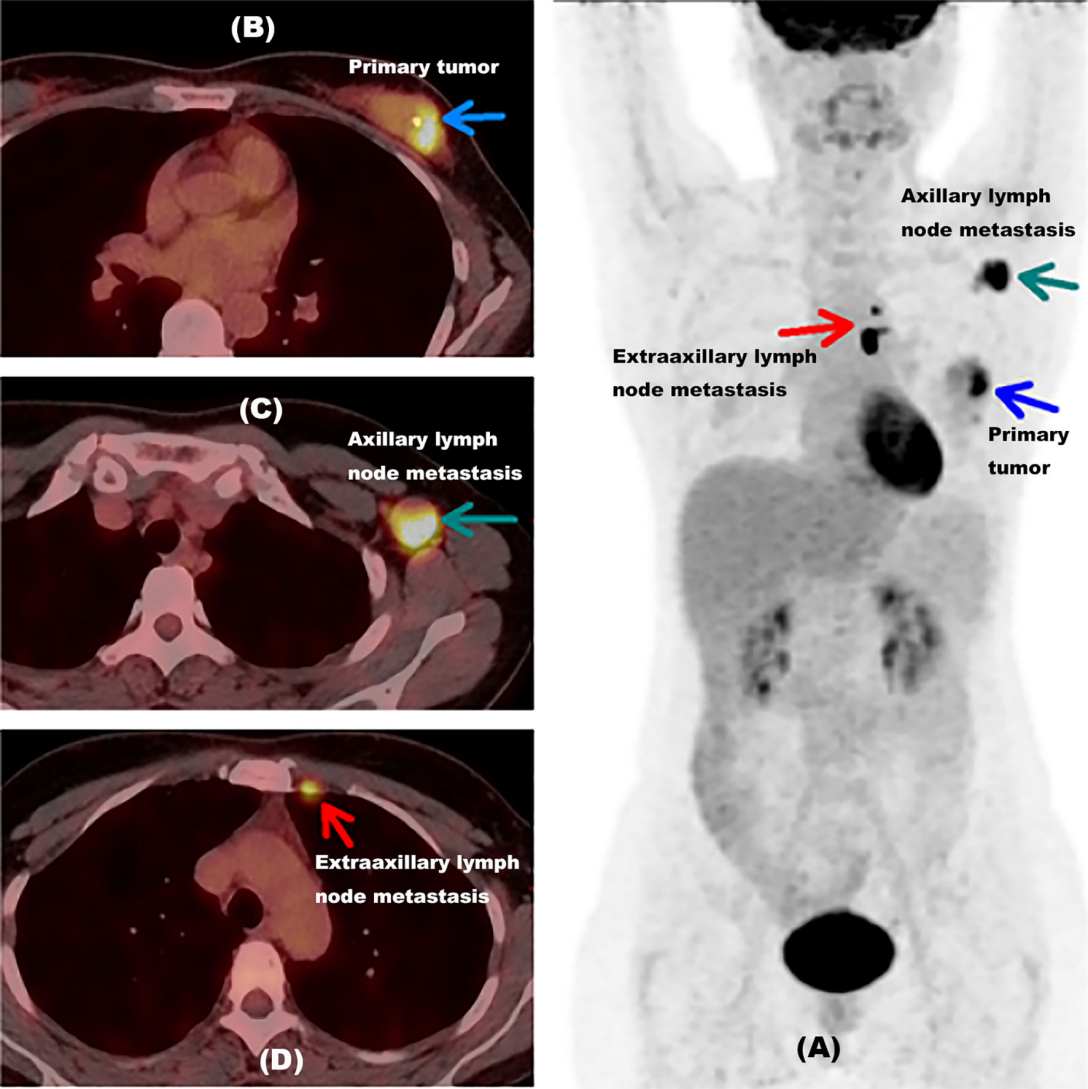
Example for a newly diagnosed breast cancer patient with unsuspected extraaxillary lymph node metastasis detected by 18F-FDG PET/CT inspection. (A) A MIP picture of a 48-year-old newly diagnosed breast cancer woman detected by an 18F-FDG PET/CT inspection with the proportion of ER at 1%. The blue arrow showed the primary tumor of this patient. The green arrow indicated axillary lymph node metastasis in the left armpit. The red arrow presented extraaxillary lymph node metastasis in the left internal mammary near breastbone. (B) PET/CT fusion image of this woman with primary tumor. The blue arrow showed the primary tumor of this patient. (C) PET/CT fusion image of this woman with axillary lymph node metastasis. The green arrow indicated axillary lymph node metastasis in the left armpit. (D) PET/CT fusion image of this woman with extraaxillary lymph node metastasis. The red arrow presented extraaxillary lymph node metastasis in the left internal mammary near the breastbone. ER, estrogen receptor; MIP, maximum intensity projection, with the proportion of ER lower than 25%.
